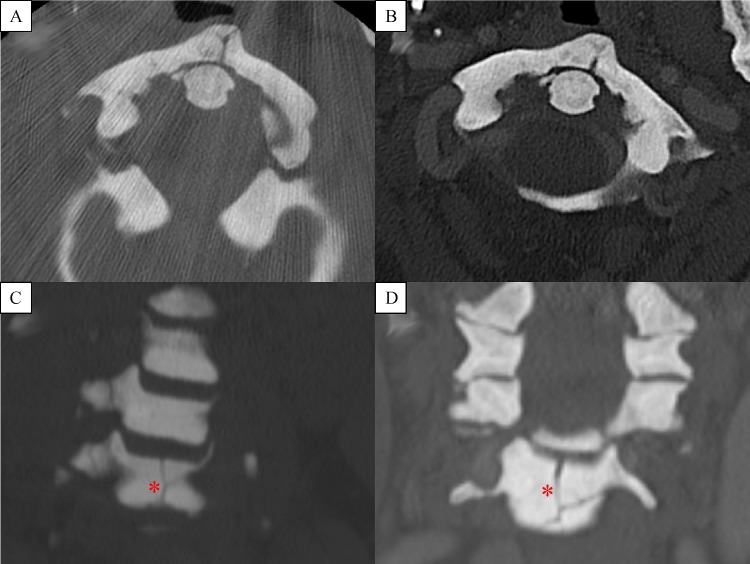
Axial CT cervical spine sequences demonstrate healing of the C1 anterior arch fracture at the time of injury (A: 2011) in comparison to four-year follow up images (B: 2015).
